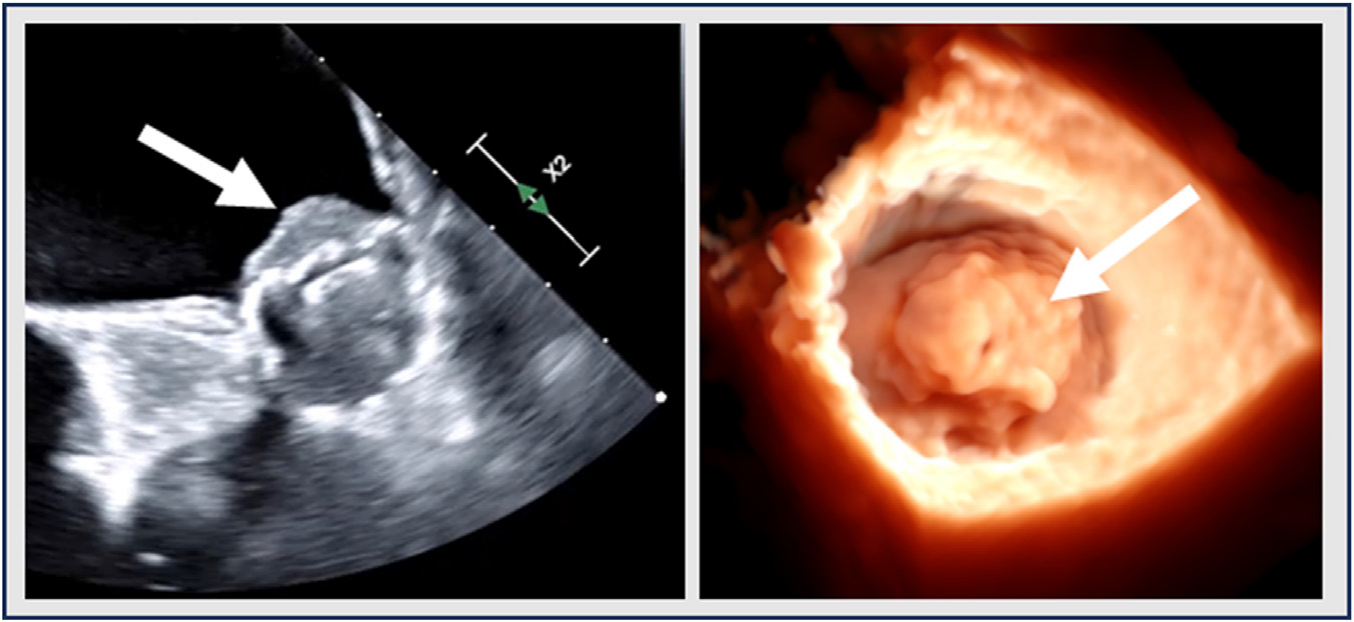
Device-related thrombus (DRT). The 2D and 3D TEE images of DRT attached to the LA surface of a Watchman 2.5 device Abbreviations: 2D, two-dimensional; 3D, three-dimensional; LA, left atrial; TEE, transesophageal echocardiography.
